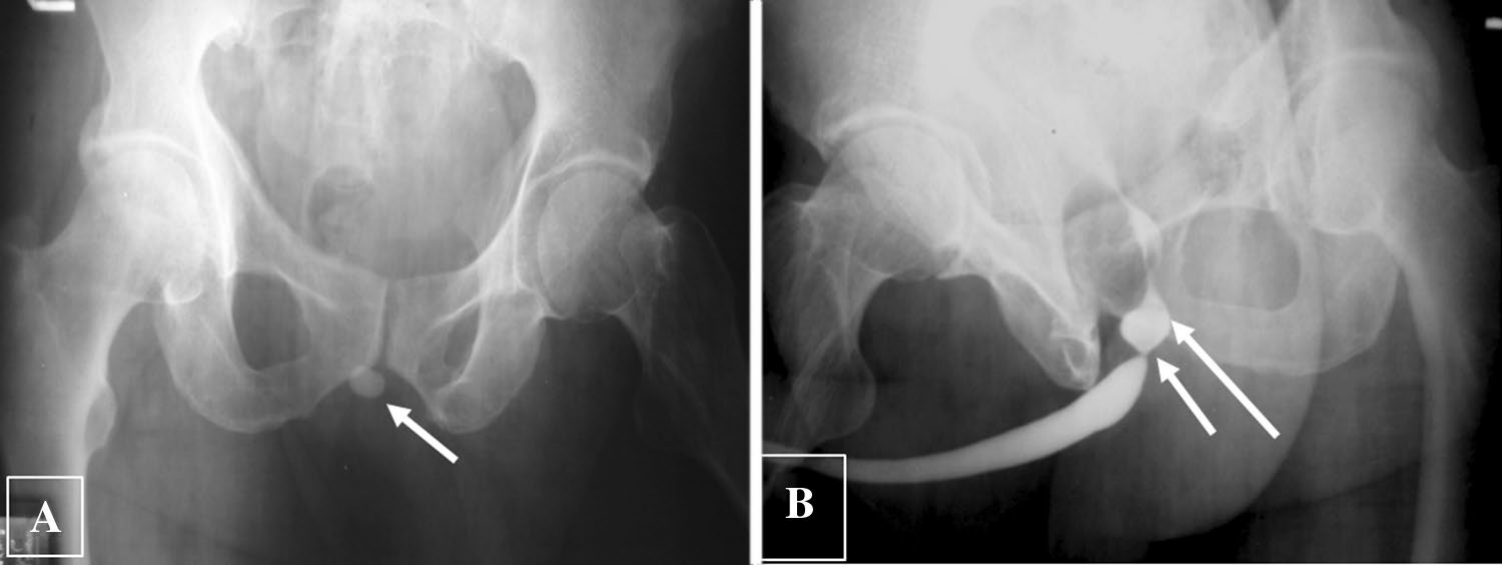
Urethral calculus. a Plain abdominal radiograph demonstrates a urethral calculus close to pubic symphysis (arrow). b Retrograde urethrogram depicts the urethral calculus as a rounded filling defect (long arrow) within the urethra proximal to a stricture (short arrow)
